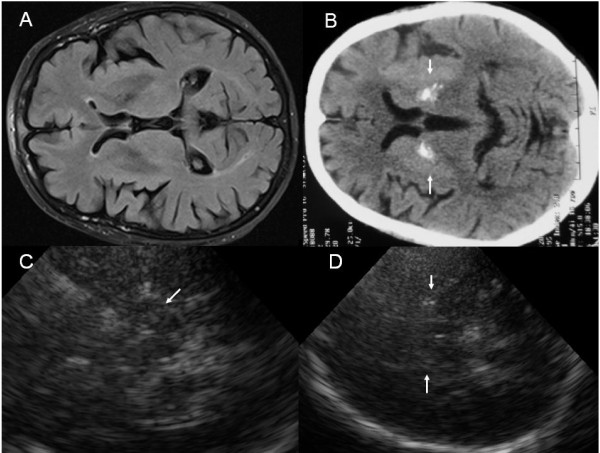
Images of a patient with ET-PD-RLS, suggesting an alternative diagnosis of parkinsonism after paraclinical tests. A-cranial MRI, T2W, B-cranial CT, markedly hyperintensive signals bilaterally in the lentiform nucleus (white arrows), C-TCS, mesencephalic plane, moderate SN and red nucleus hyperechogenicity (white arrow), D-TCS, diencephalic plane, bilaterally markedly hyperechogenic lentiform nuclei (white arrows).
